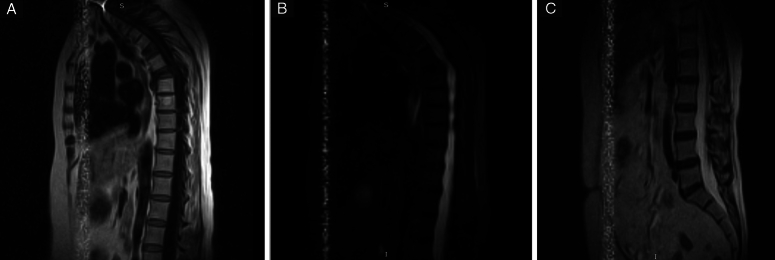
Magnetic resonance imaging shows: T1, compression fracture in T5 and T7 with backward displacement of the vertebral body in (A). T2, compression of the thoracic spinal cord (B). T1, no changes or injury in the lumbar area (C).
